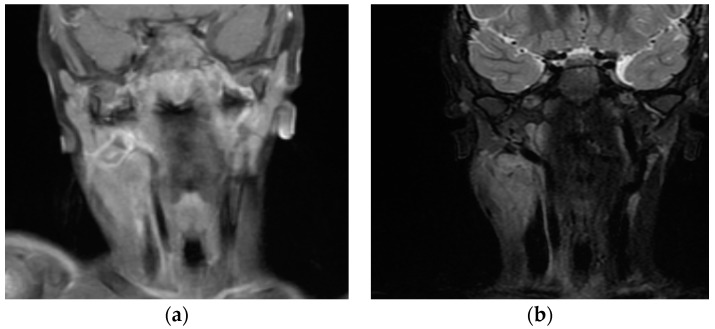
Magnetic resonance imaging (MRI) of the neck. (a): Right anterolateral multiple polycyclic lymph nodes with ill-defined contours and tendency to confluence. Intense enhancement of the adjacent sternocleidomastoid. Displacement of the vascular bundle of the neck and internal jugular vein compression. (b): Enhancement of parapharyngeal and retropharyngeal tissues without fluid collection and with preserved respiratory space. Multiple bilateral enlarged lymph nodes in parapharyngeal and retropharyngeal space. Minimum contrast enhancement of the atlantoaxial joint.
